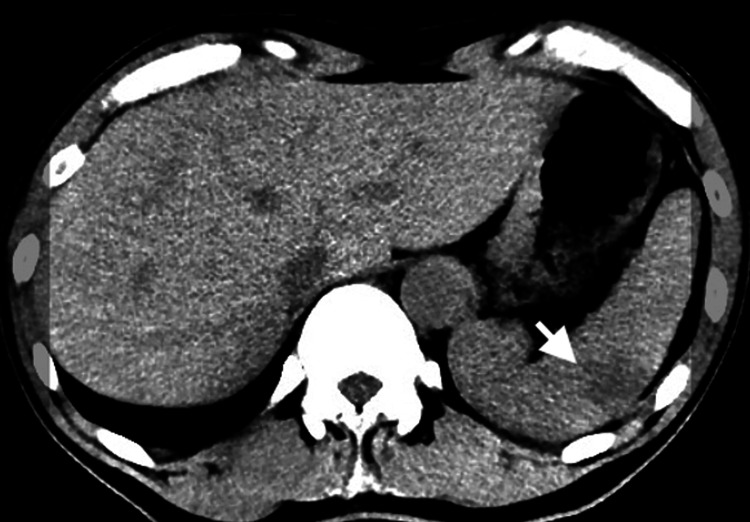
Splenic infarction on abdomen CT Complications of *Abiotrophia defectiva* infective endocarditis.
